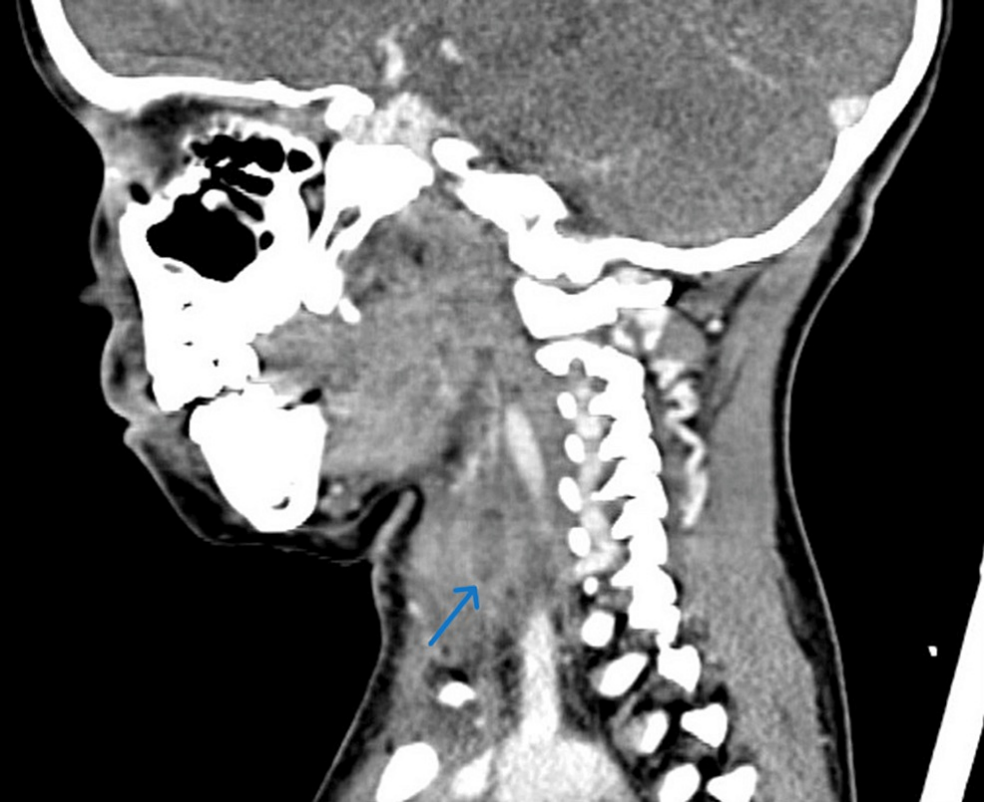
Sagittal view of contrast-enhanced computed tomography of the neck
